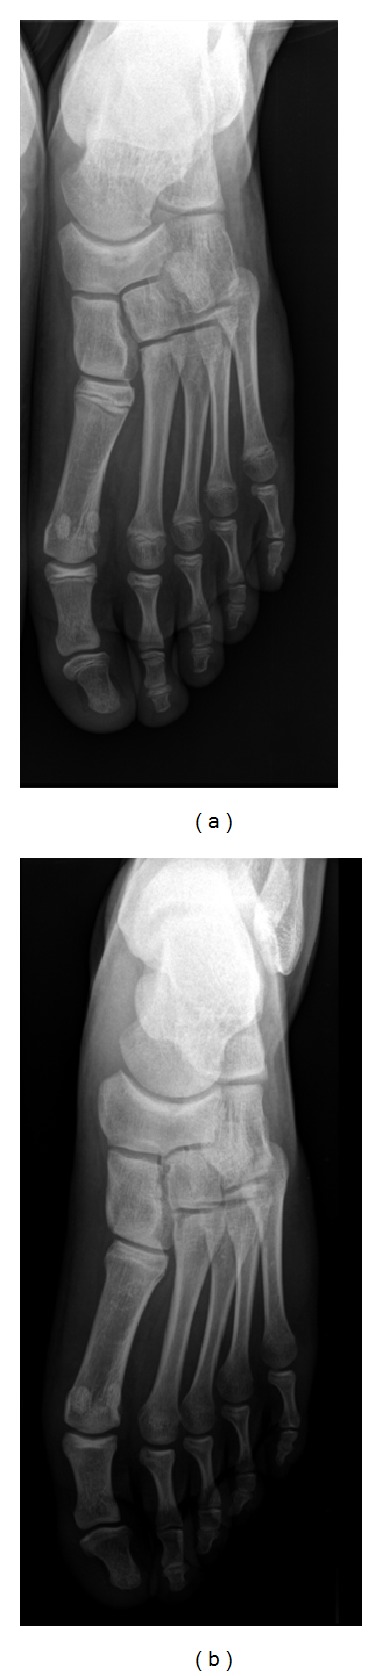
Radiographs of the left foot at 4-month (a) and 2-year (b) follow-up consultations, showing a favorable radiologic evolution.
